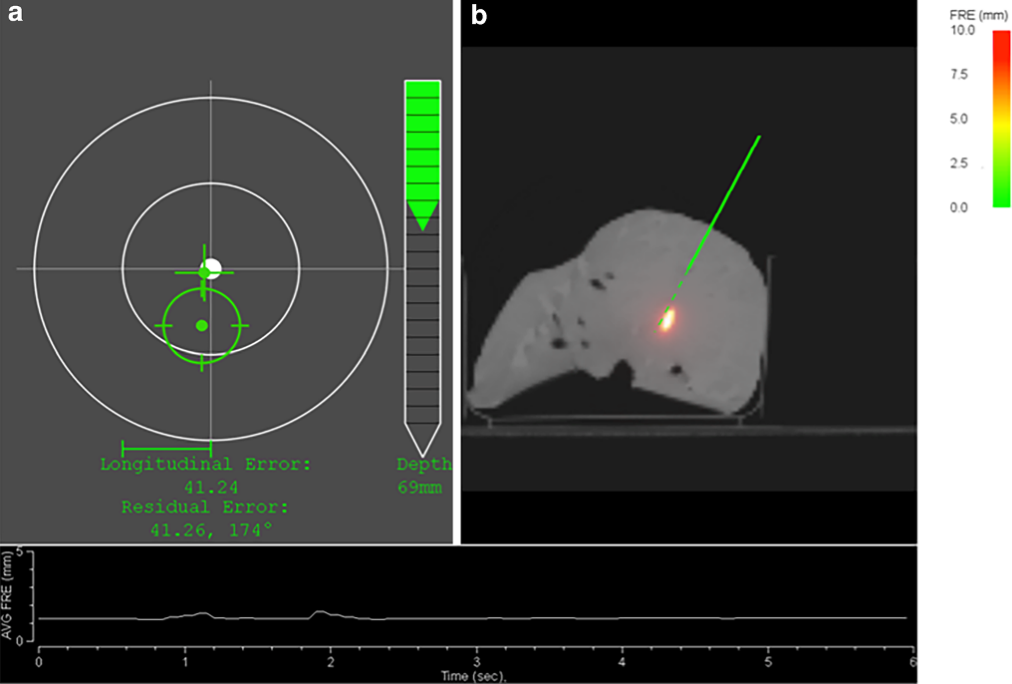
When the needle is close to the planned entry, the system displays a cross-hair viewer (a), where the needle tip is represented by a *cross* and the needle shaft by a *circle*. A depth bar guides the user towards the target. Displayed in b is a resliced CT, showing the current path of the needle. The curve at the bottom of the screen displays the amplitude of patient deformation
